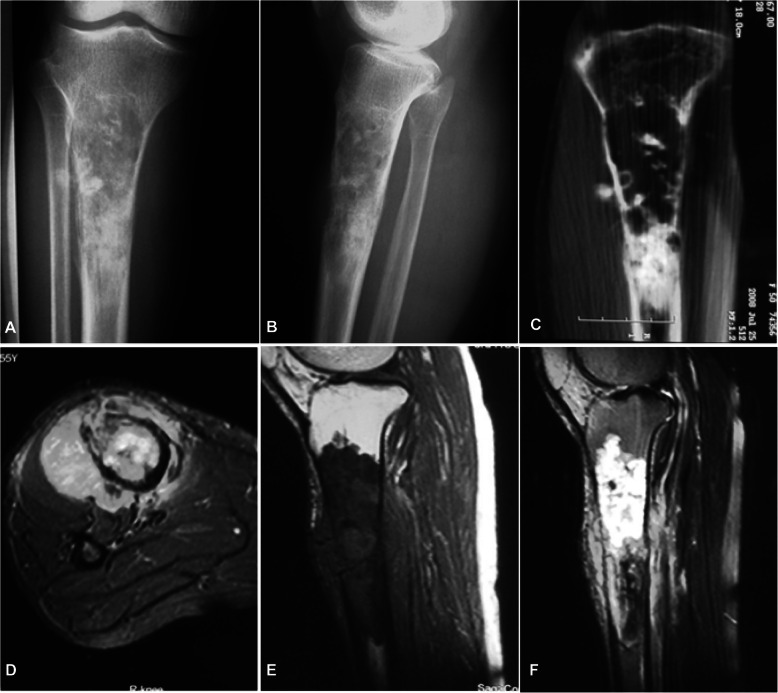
A 55-year-old woman had a mass at the tibia for half a year and was diagnosed with dedifferentiated chondrosarcoma. a, b Plain radiograph revealed bone destruction at the proximal segment of right tibia with ill-defined margins, irregular circular and patchy calcification, and periosteal reaction. c Coronal computed tomography reconstruction showed bone destruction, irregular and circular calcification. d-f. Magnetic resonance imaging in STIR sequence (d), T1WI (e) and fat-suppression T2WI (f) showed long T1 and long T2 signal with low signal in calcification and ossification. The bone destruction and typical circular calcification of the tumor cartilage are typical for chondrosarcoma
